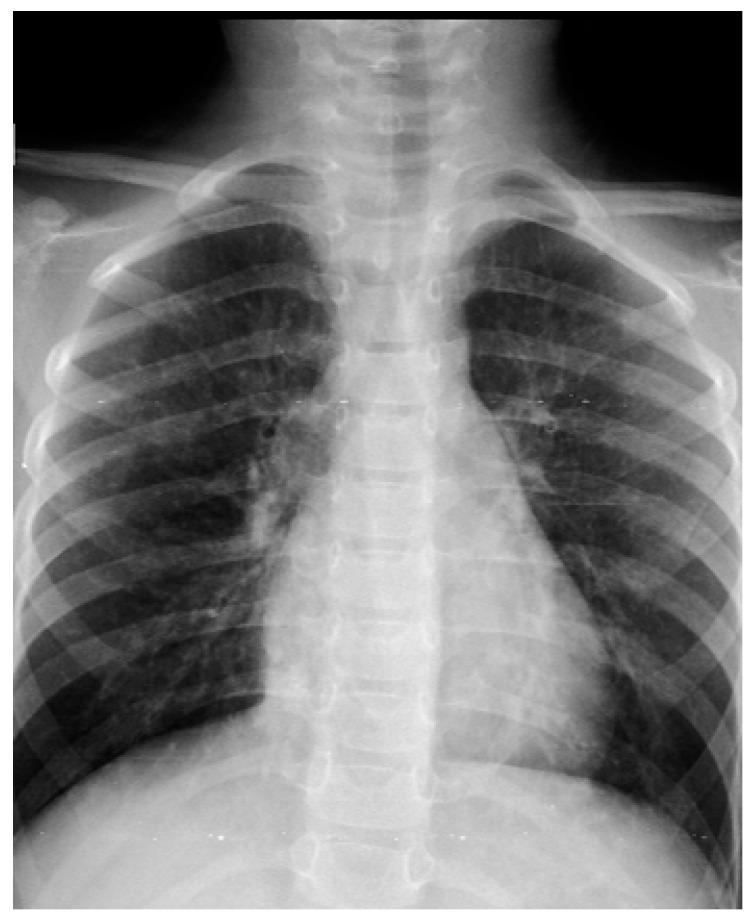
Plain chest radiography demonstrated bilateral perihilar peribronchial thickening with mild hyperinflation, without focal lobar consolidation or pleural effusion—findings consistent with chronic/recurrent airway inflammation.
